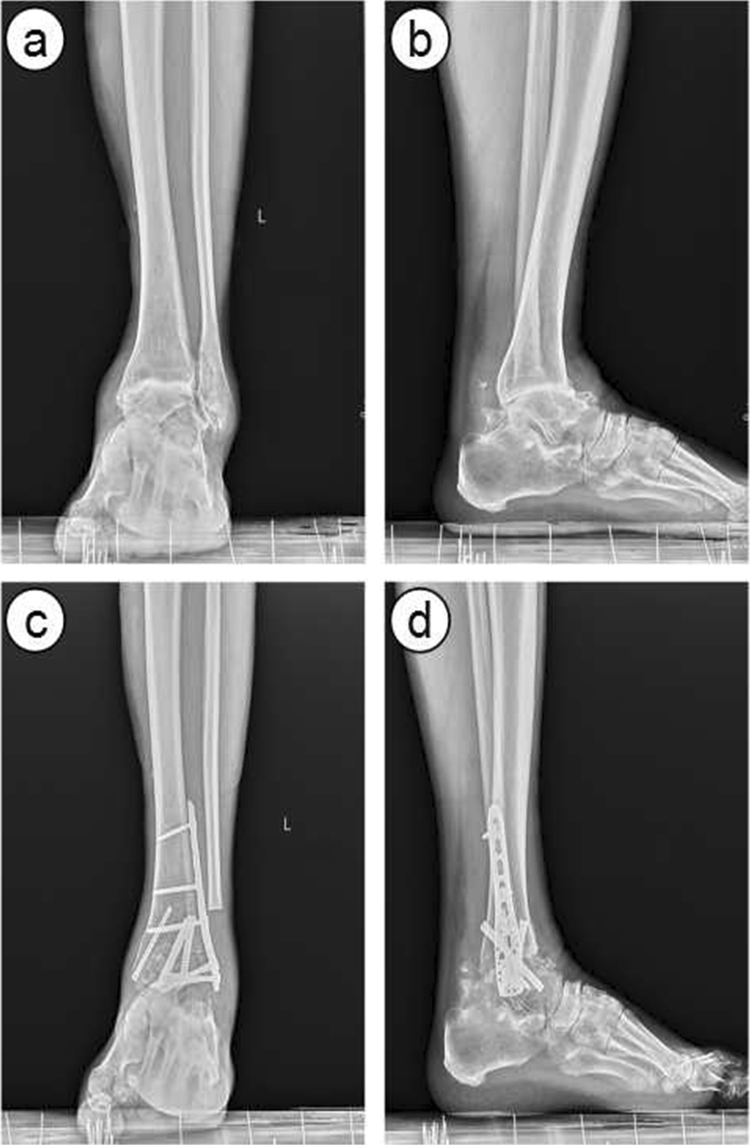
Radiographs showing a 45 yrs patient with end-stage ankle arthritis underwent plate internal fixation. (a) and (b) pre-operative anteroposterior and lateral radiographs, (c) and (d), post-operative 3 month anteroposterior and lateral radiographs.
